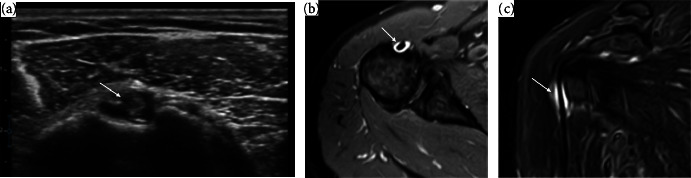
US and MRI findings of 50-year-old female patient with 4-year hemodialysis duration, presenting with shoulder pain for 6 month. (a) US scan transverse view of biceps tendon shows a hypoechoic area around the biceps tendon (arrow) denoting tenosynovitis. (b & c) MRI shoulder axial &coronal STIR images revealed mild amount fluid SI within synovial sheath of biceps tendon (arrow) denoting biceps tenosynovitis.
